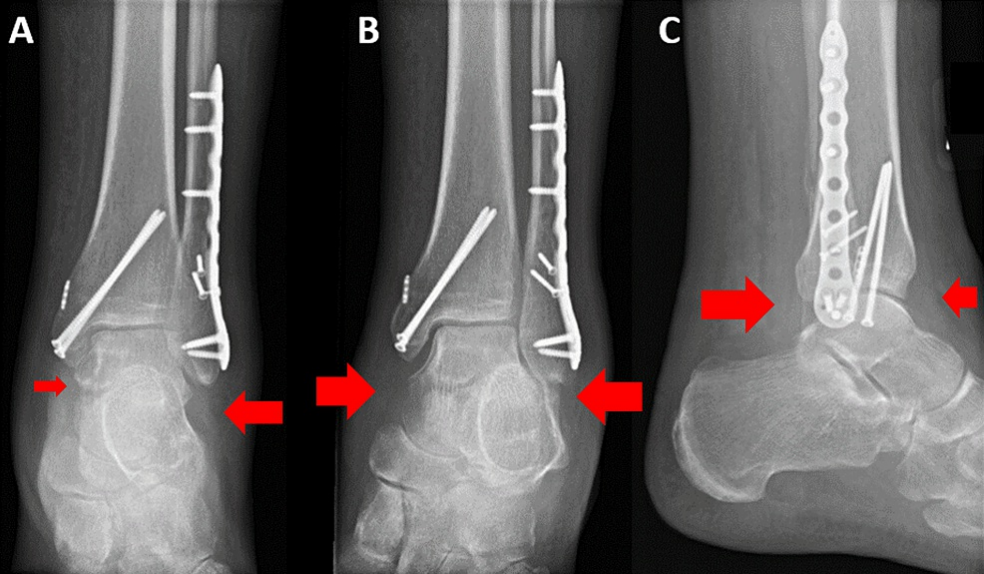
Radiographs taken in the emergency department of the patient's left ankle with anteroposterior (A), mortise (B), and lateral views (C) with red arrows indicating soft tissue swelling.
